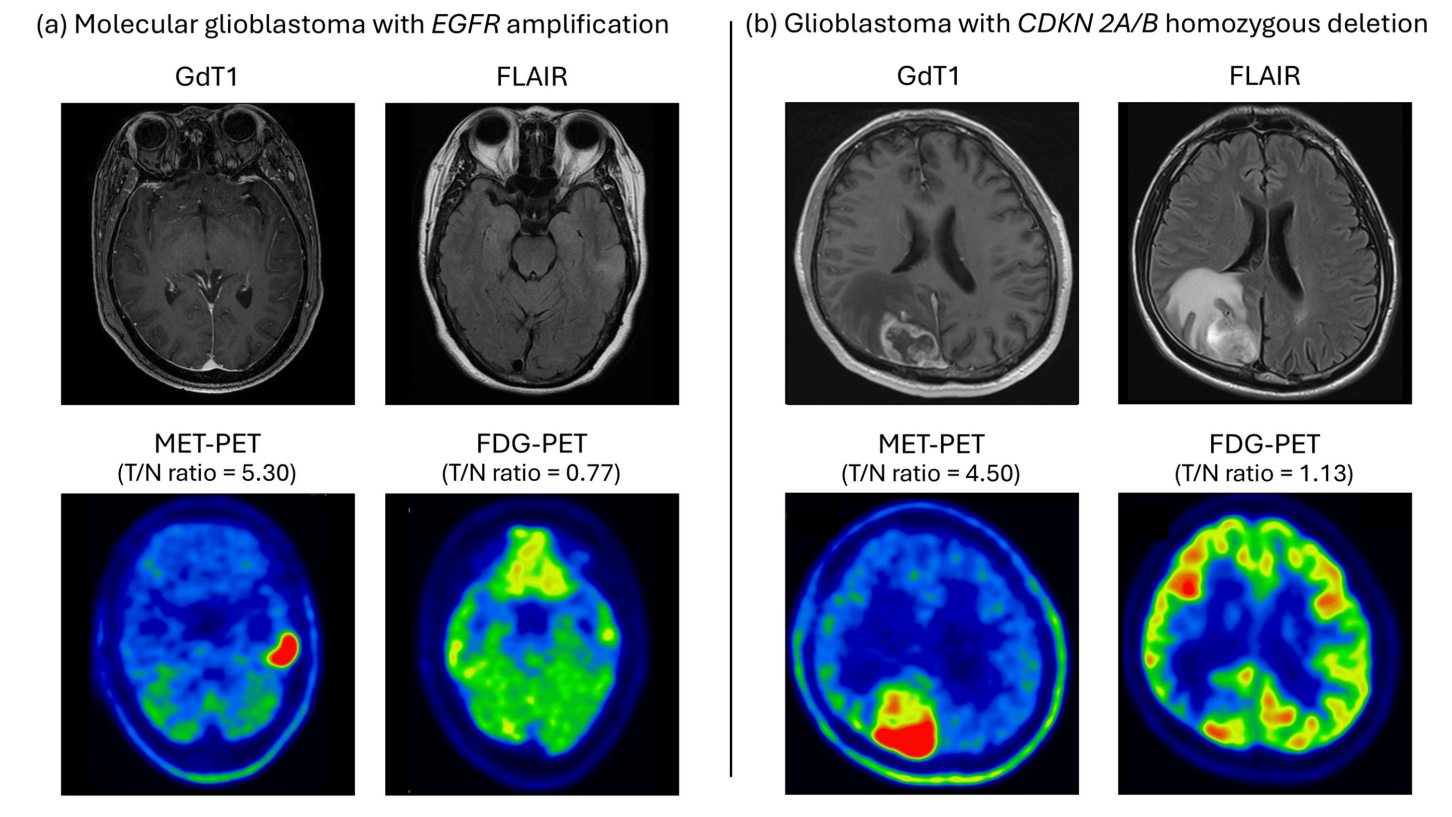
Representative cases of molecular glioblastoma with EGFR amplification and IDH-mutant, WHO grade 4 astrocytoma with CDKN2A/B homozygous deletion (HD) (a) The molecular glioblastoma case with the lesion observed in the left temporal lobe, no contrast enhancement on GdT1 MRI, hyperintensity on FLAIR, and reduced glucose uptake on FDG-PET (T/N ratio = 0.77). The lesion had intense methionine uptake on MET-PET (T/N ratio = 5.30). (b) The IDH-mutant, WHO grade 4 astrocytoma was associated with a mass lesion in the right occipital lobe, with enhancement on GdT1 MRI, peritumoral edema on FLAIR, increased metabolic activity on MET-PET (T/N ratio = 4.50), and reduced glucose uptake on FDG-PET (T/N ratio = 1.13). PET, positron emission tomography; MET, l-methyl-11C-methionine; 18F-FDG, fluorine-18 fluorodeoxyglucose; MRI, magnetic resonance imaging; FLAIR, fluid-attenuated inversion recovery; T/N ratio, SUVmax, maximum standardized uptake value; ratio of SUVmax in tumors to SUVmax in normal tissues Note: All images shown in this figure are from cases diagnosed and treated at the author's institution.
